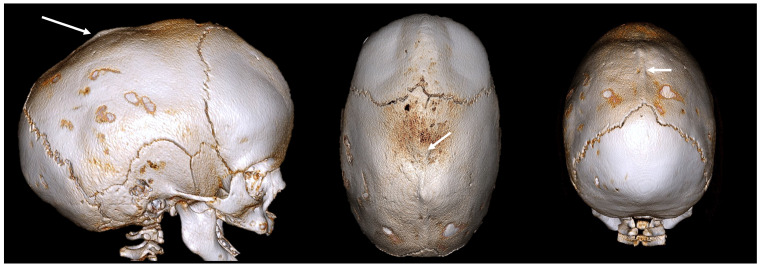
A 3D CT image of a sagittal CRS (scaphocephaly) with an elongated skull, a prominent forehead, and occipital bulging. The white arrow indicates an abnormally fused suture.
